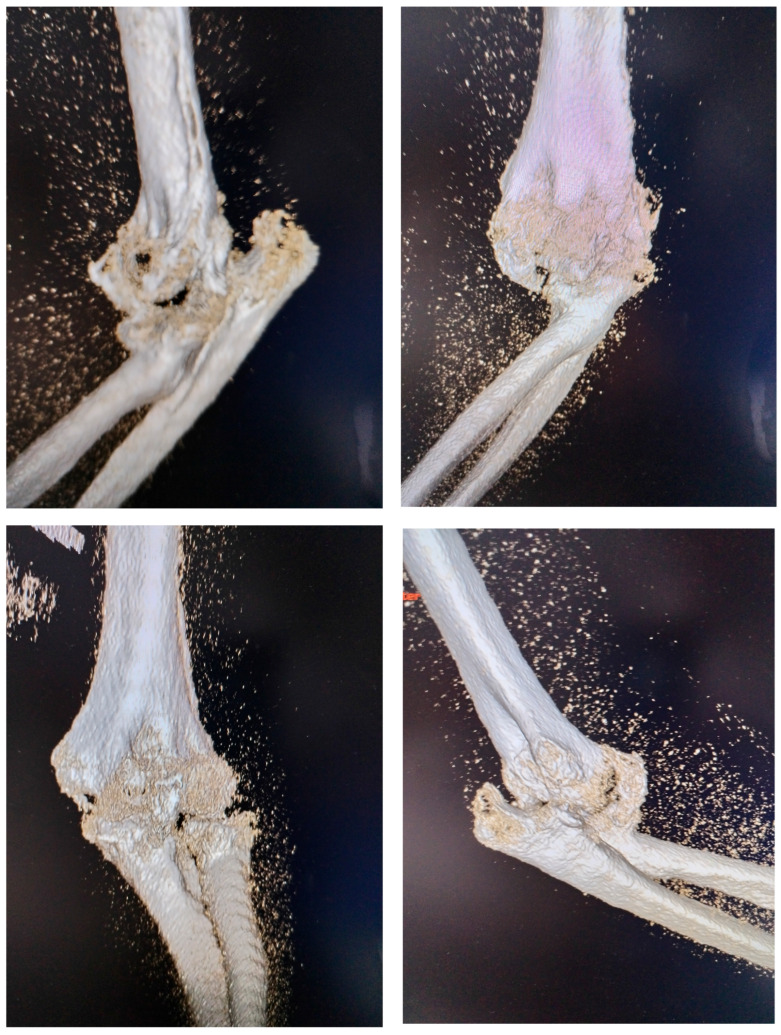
3D CT aspect with visualisation of dislocation and presence of a massive heterotopic bone inside the joint.
